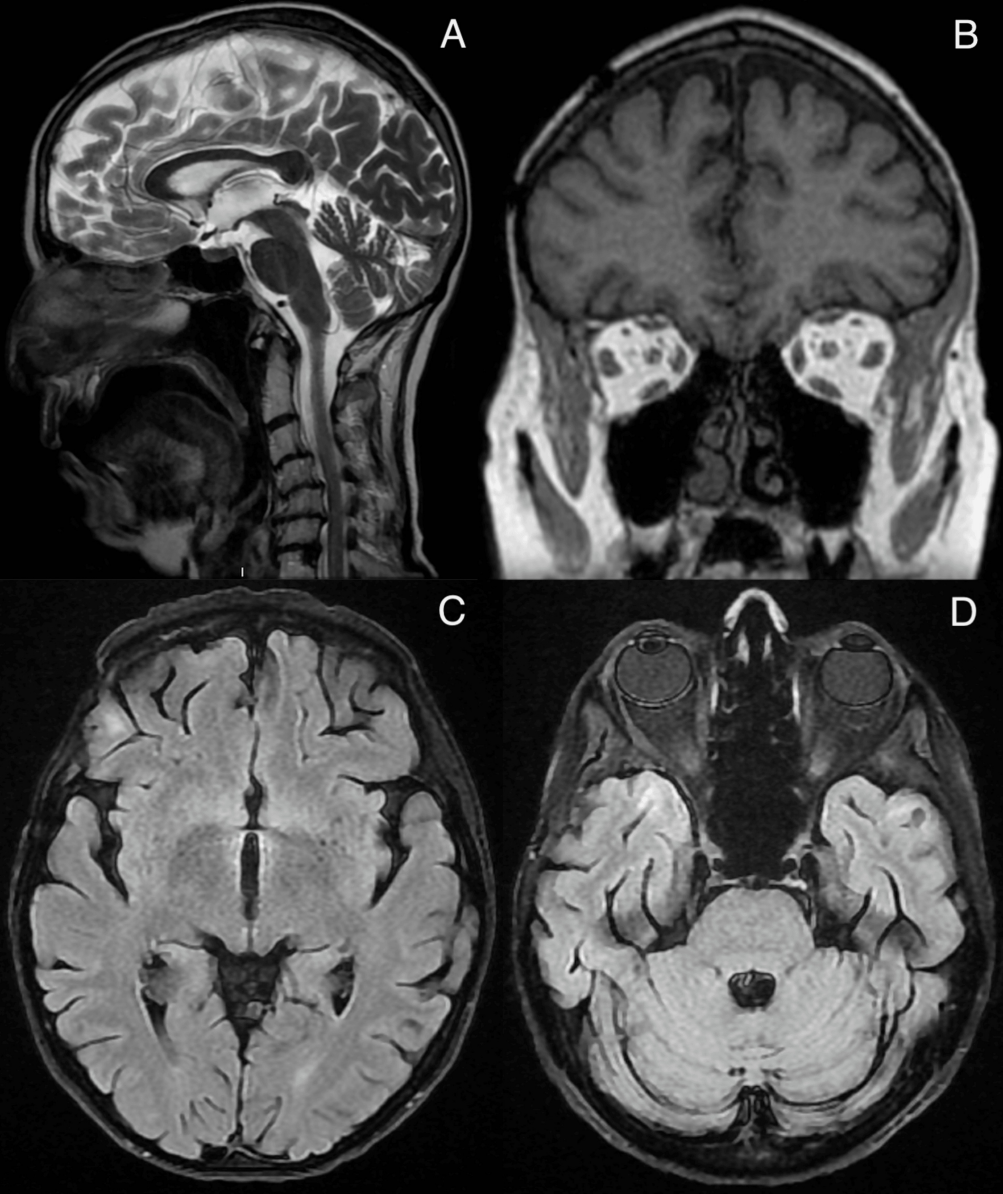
MRI brain sequences demonstrating no abnormal signal or structural lesions suggestive of active neuropsychiatric lupus or metastatic disease. A: sagittal plane, demonstrating no evidence of acute intracranial pathology at the level of midline. B: coronal plane, demonstrating no evidence of acute intracranial pathology at the level of the frontal lobes. C: axial plane, demonstrating no evidence of acute intracranial pathology at the level of the cerebral cortex and basal ganglia. D: axial plane, demonstrating no evidence of acute intracranial pathology at the level of the tentorium cerebelli.
